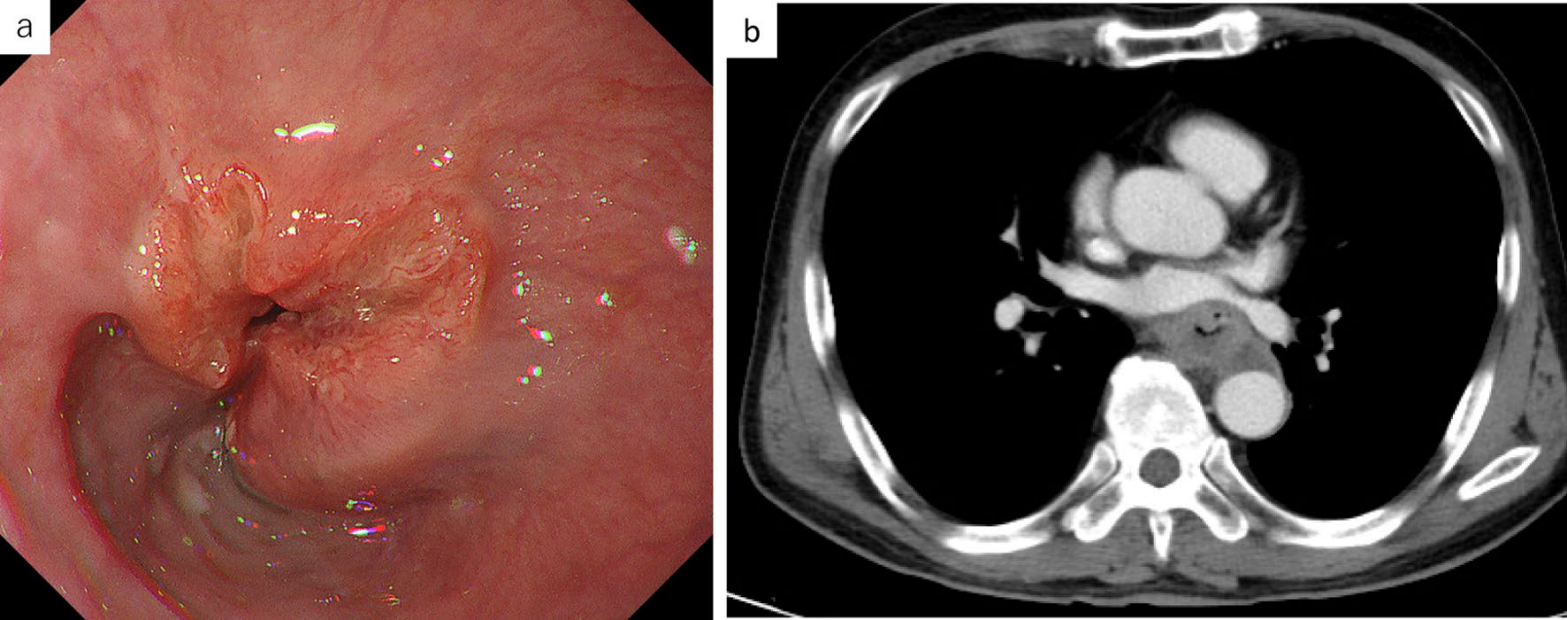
Pretreatment imaging tests. a Esophagogastroduodenoscopy showed a sub-circumferential type-3 tumor in the posterior wall of the middle thoracic esophagus. b Computed tomography showed esophageal wall thickness of the middle thoracic esophagus and the anterior thoracic paraaortic lymph node in contact with the descending aorta
